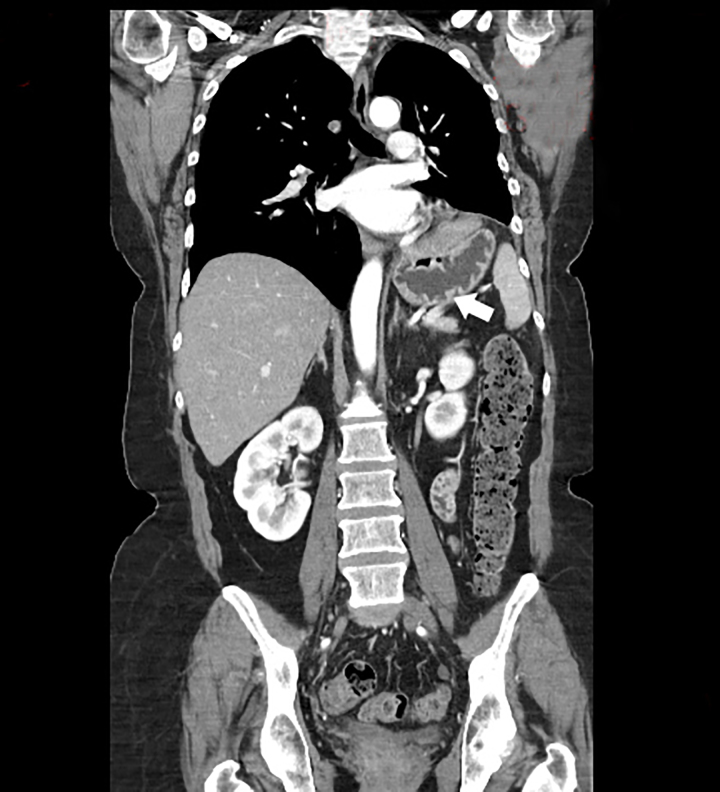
A control chest CT shows gastric body in normal anatomical position. *CT,* computed tomography
